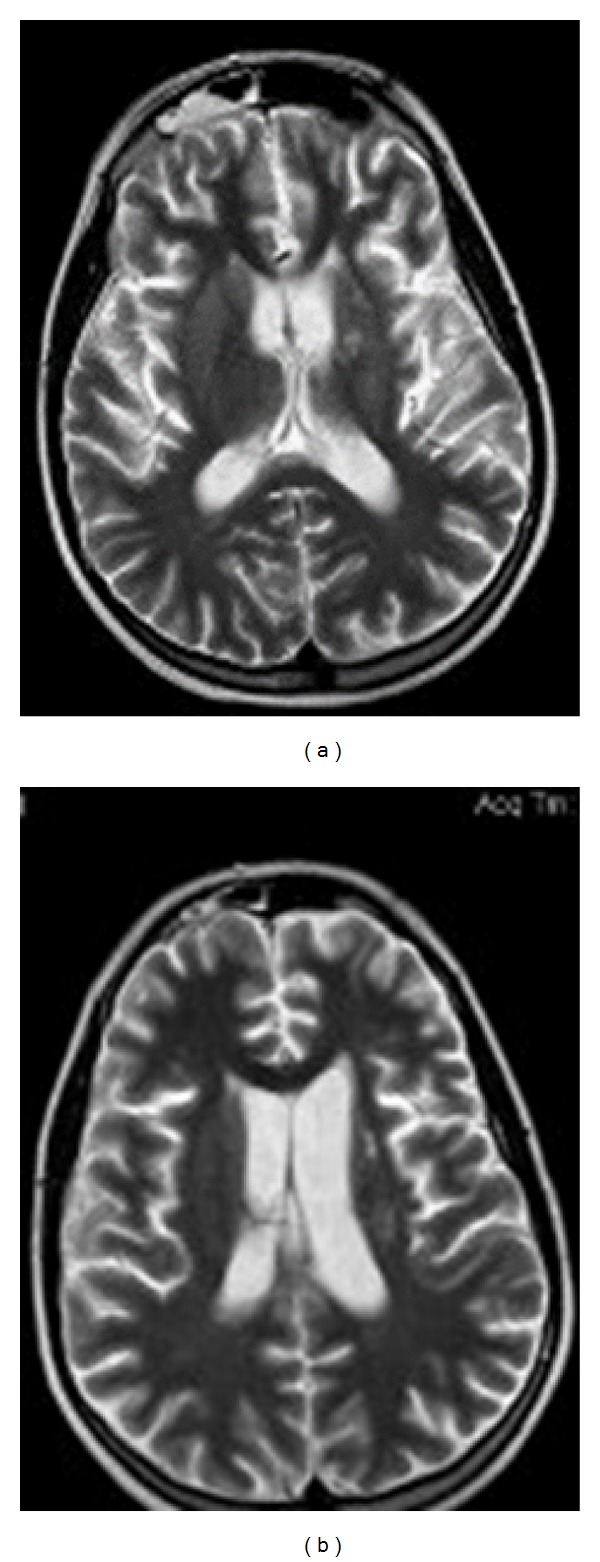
T2-weighted images reveal hyperintense lesions over the left internal capsule (a) and over corona radiata (b).
